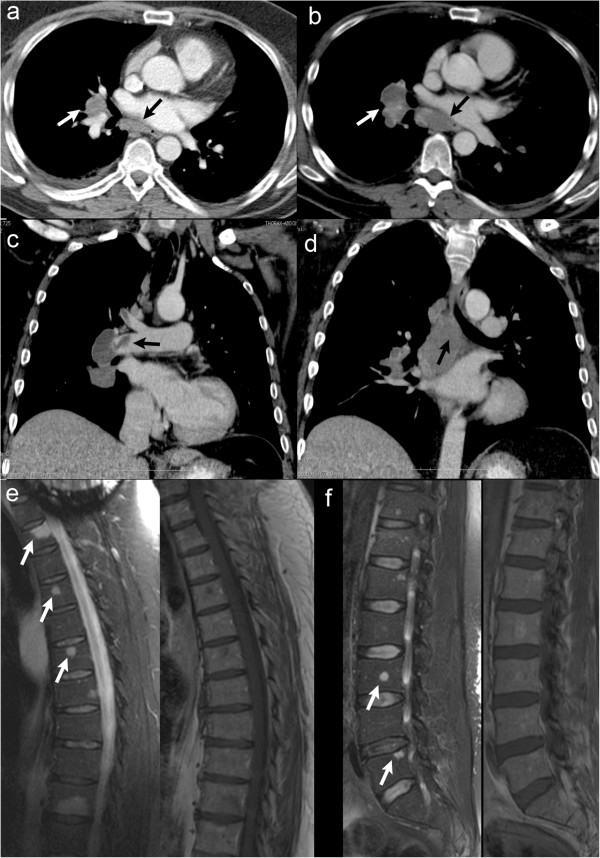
Staging with CT of the thorax and spinal MRI. Contrast enhanced CT of the thorax (a-d) revealed rapidly growing (a in 05/2011, b-d in 08/2011) hilar (white arrow in a and b) and infracarinal (black arrow in a,b,d) lymph node metastases with infiltration of the right pulmonary artery. Additionally thromboembolic material was found in branches of both pulmonary arteries (arrow in c). MRI of the thoracic (e) and lumbar (f) spine showed multifocal osseous metastases (arrows).
